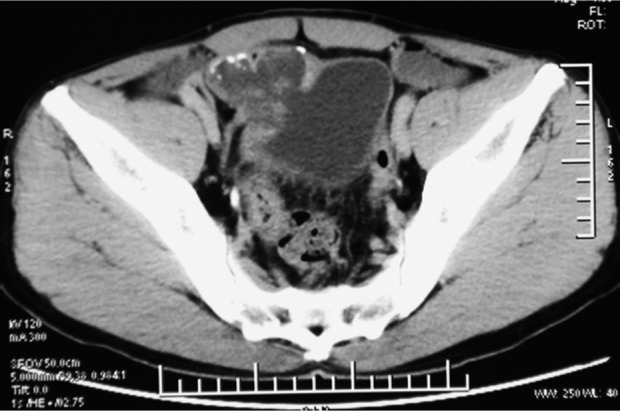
CT scan shows an irregular slightly higher density. The largest cross-section diameter was 5.2 cm×4.8 cm and its CT value was slightly higher than the intravesical CT value. There was some punctate calcification on the edge and inside the hyperdense area.
